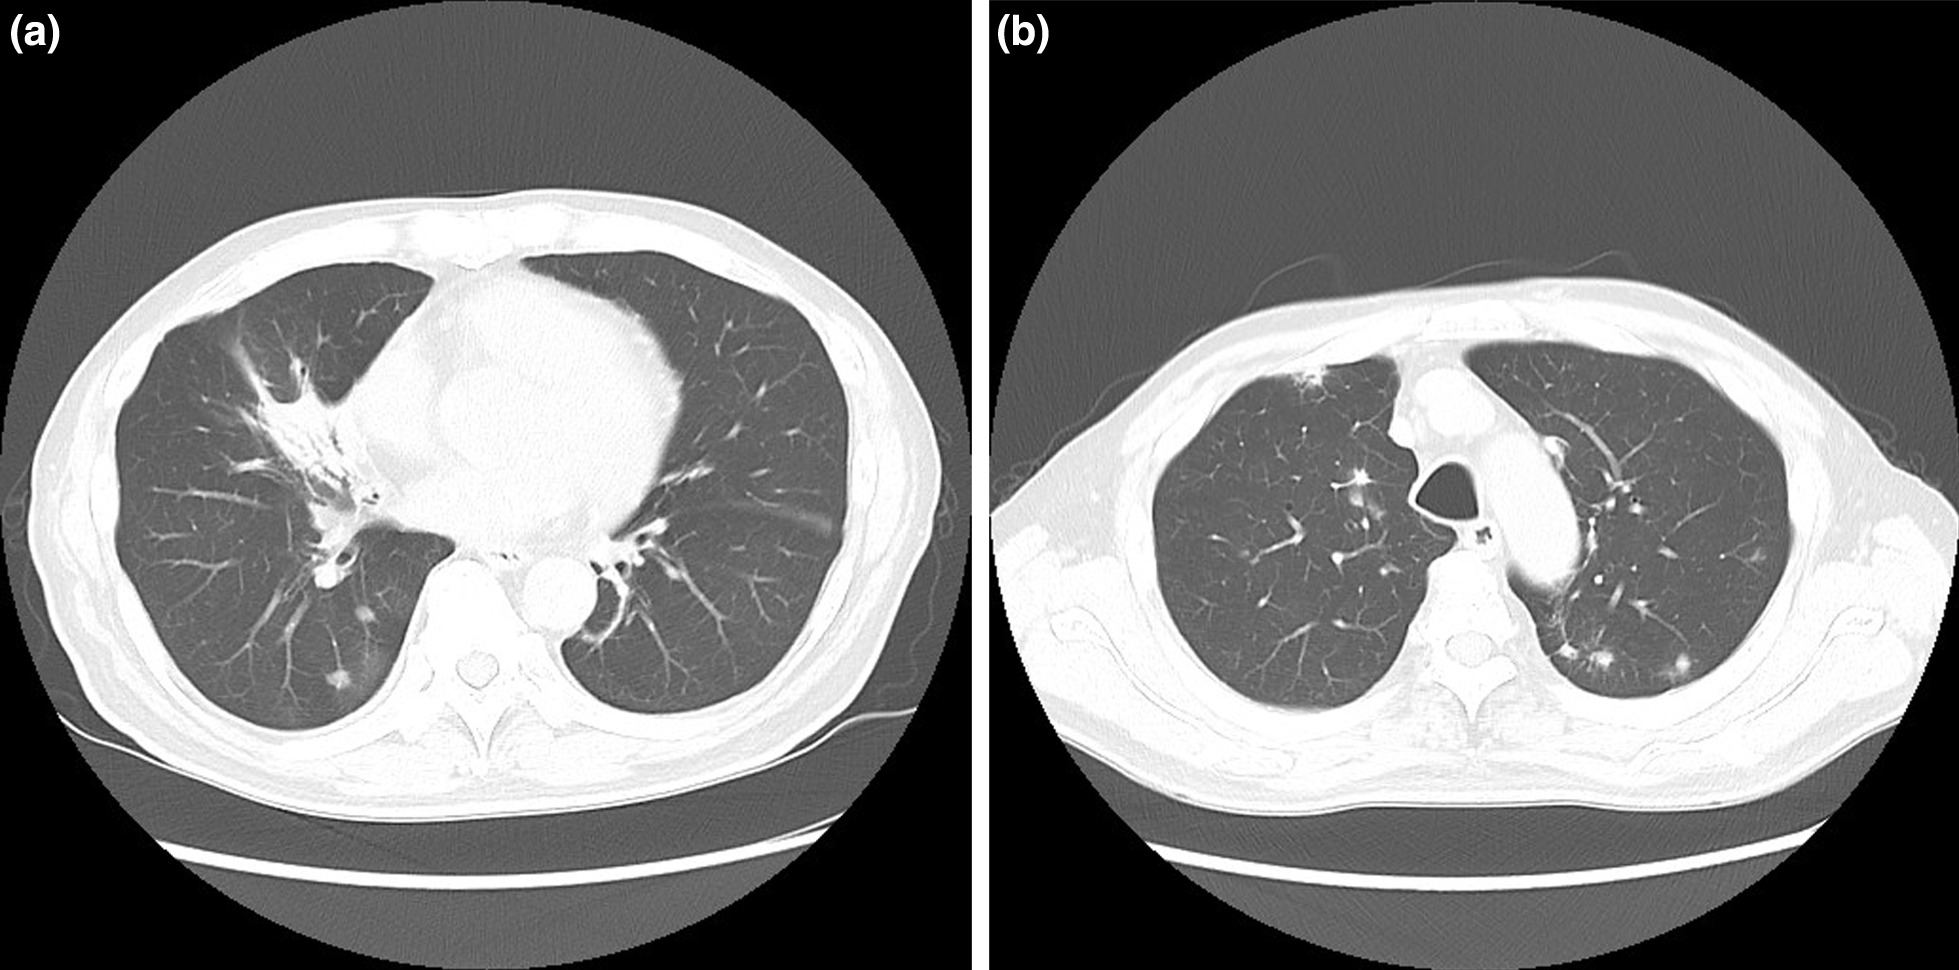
Chest CT view at the third month of PSL treatment (30 mg/day). a, b Chest CT showing a shrinking middle lobe hilar mass and multiple nodular shadows in both lungs
